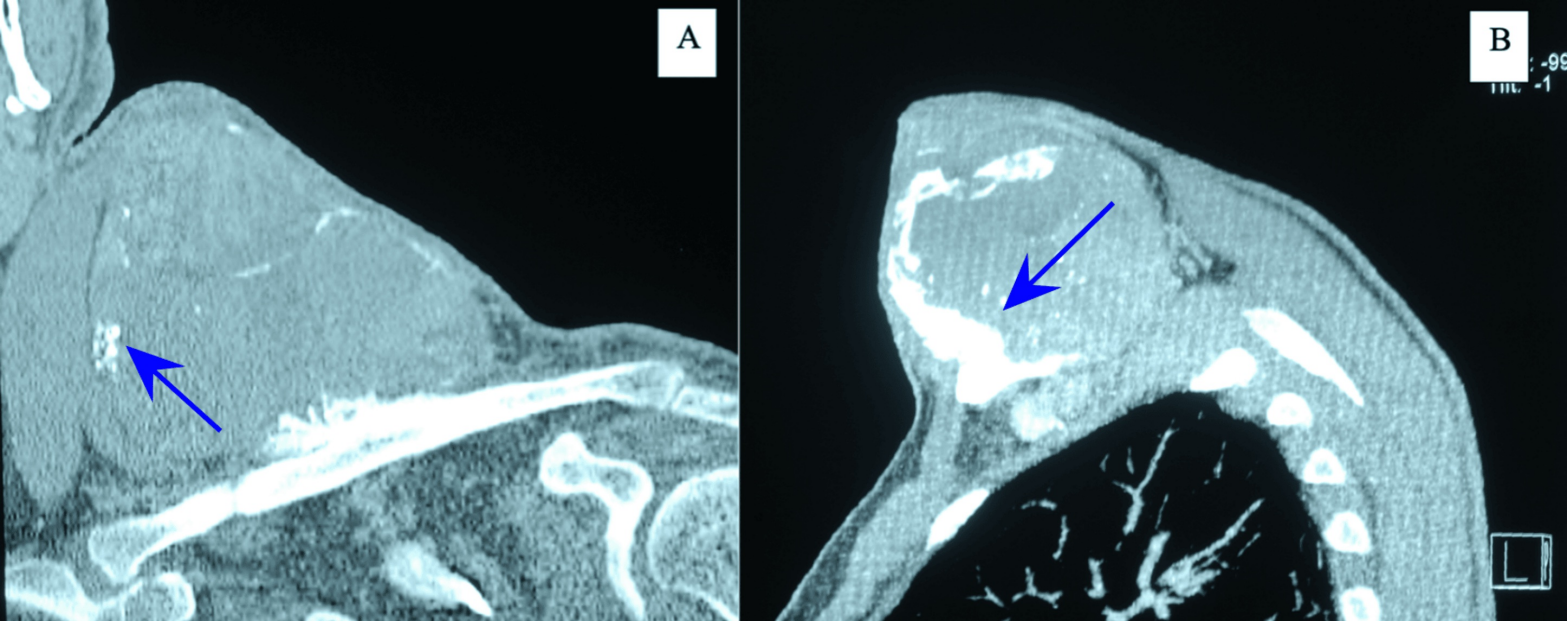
CT scan of the tumor CT scan of the tumor shows a juxtacortical, well-defined, lobular lesion with invasion of the surrounding soft tissues and mineralization along its margins. A: coronal view; B: sagittal view
